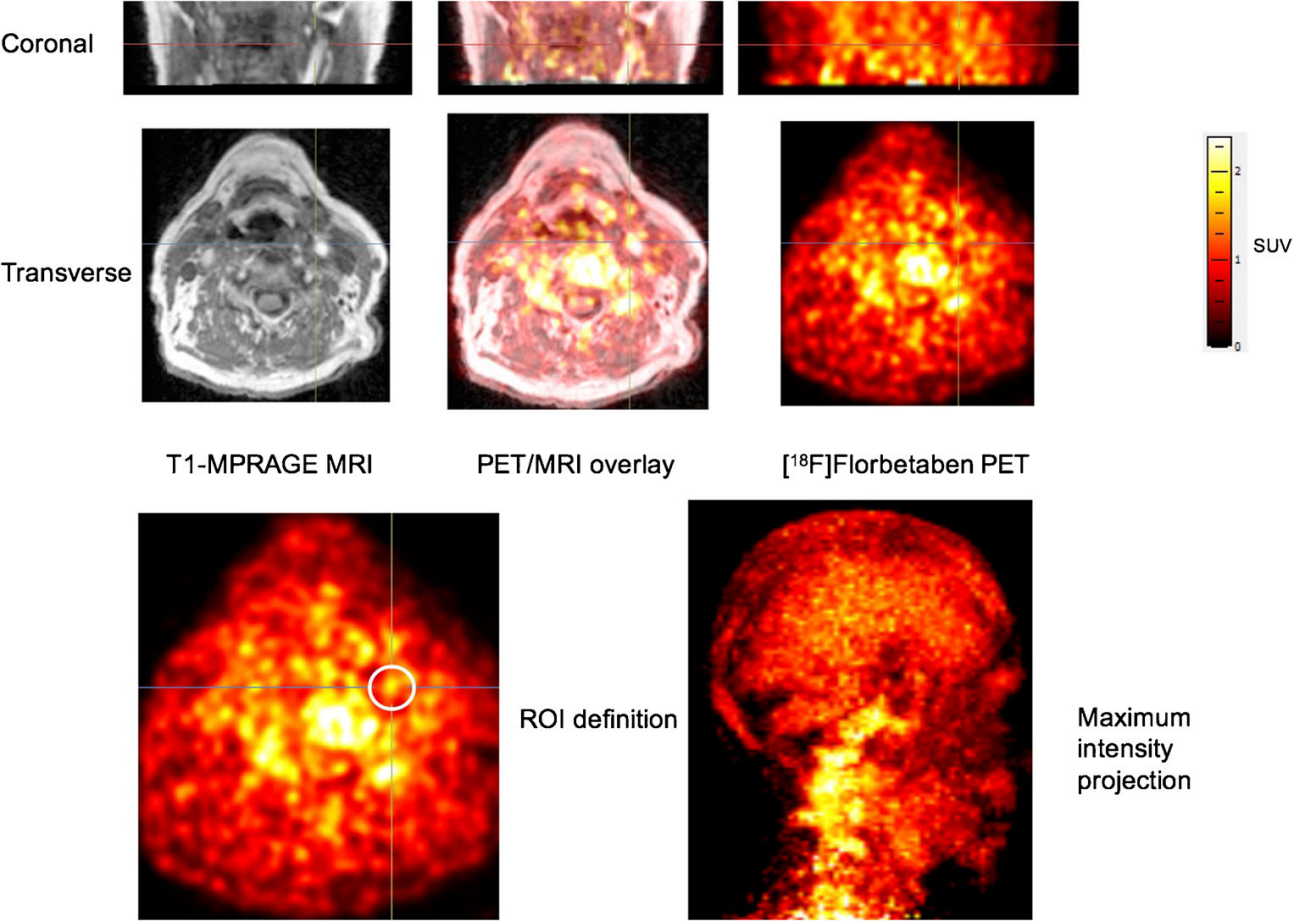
Focally increased ^18^F-florbetaben uptake in the left common carotid artery. *Top set of images* Coronal and transverse T1-W MR images, fused PET/MR images and PET-only images. *Bottom pair of images* Transverse PET slice with a ROI (*white circle*) placed around the lumen of the left common carotid artery including the focally increased ^18^F-florbetaben uptake, and a PET maximum intensity projection image with the carotid artery hot spot. Both visual and semiquantitative analyses revealed higher tracer uptake in the left than in the right common carotid artery (SUV_mean_ left 1.33, right 1.06; SUV_max_ left 1.83, right 1.22, ROI for the right common carotid artery not shown). A standard ROI within the lumen of the left jugular vein was used (not shown) for estimation of the ^18^F-florbetaben blood pool activity for calculation of the target-to-background ratio
